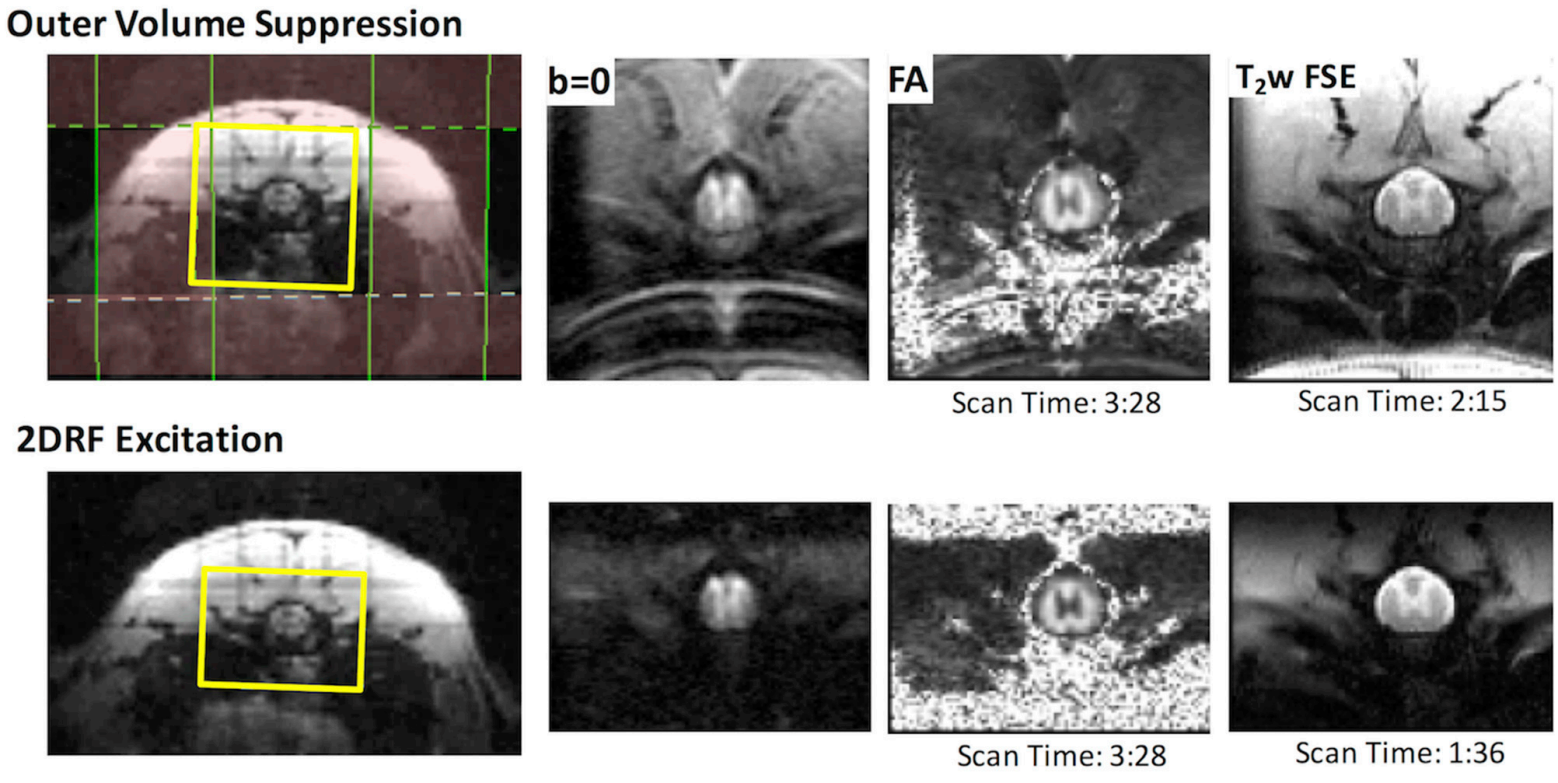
Reduced Field of View DWI and T_2_-weighted Imaging. rFOV using outer volume suppression (top) or 2DRF (bottom) provided high-quality images of the thoracic spine. Fold-over and chemical shift artifacts were evident in the OVS, which includes a separate fat-suppression module. The 2DRF with a slightly smaller FOV but an identical resolution offered similar SNR, minimal chemical shift artifacts (without separate fat suppression), and slight improvement in EPI distortion as shown by the *b* = 0 images and FA maps. In a T_2_-weighted fast spin echo, the smaller FOV allowed a reduction in acquisition time with a comparable SNR.
